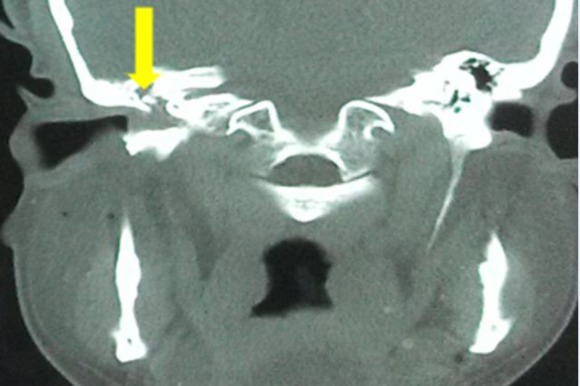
Computed tomography temporal bone (coronal view) showing soft tissue density mass in right tympanic cavity (yellow arrow) extending to the external auditory canal
